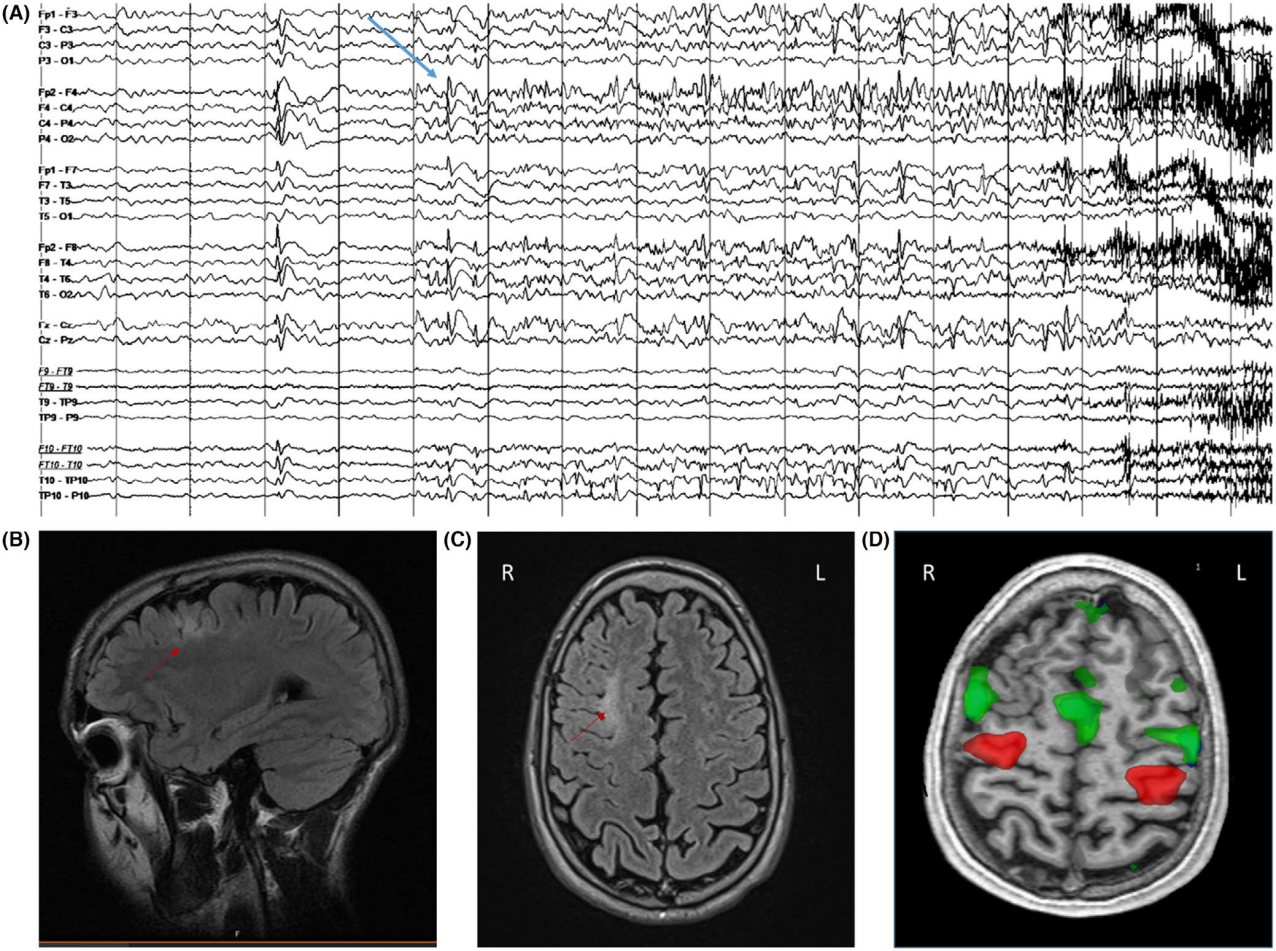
Phase I work‐up in a 24‐year‐old right‐handed male with a family history of epilepsy in his paternal uncle and nephew. Epilepsy onset was at age 7 years, initially with focal impaired consciousness seizures during childhood characterized by left‐sided facial twitching and leftward gaze deviation which no longer occurred after the age of 9. Subsequently, all seizures were focal to bilateral tonic–clonic, occasionally preceded by an ill‐defined aura described as full body discomfort, occurring monthly. Seizures were recorded as episodes typically occurring out of sleep and rarely preceded by an ill‐defined aura (patient alerts observer or pushes the alarm, however, is unable to explain his symptoms) and hyperventilation, with subsequent versive head turn to the left, gaze deviation to the left, tonic posturing with Figure 4 (flexion of the right upper extremity, extension of the left upper extremity), followed by bilateral clonic activity with postictal confusion. (A) Clinical onset typically occurred 8–10 s after electrographic onset, with first ictal changes on scalp EEG characterized by spike and slow wave discharges followed by 10–12 Hz repetitive spiking over the right frontal region (with maximum negativity over F4 > Fp2 > Fz) (arrow, 16 s, 1–70 Hz bandpass, bipolar longitudinal montage with subtemporal chains). (B, C) 3 T Brain MRI showed T2/FLAIR hyperintense signal and blurring of the gray‐white matter junction at the level of right superior frontal sulcus. (D) Functional MRI showed left dominant expressive and receptive speech with accessory expressive speech within the right frontal lobe 1–2 cm inferior and lateral to the lesion (green), and left hand motor function within 1 cm posterior and lateral to the right frontal lobe lesion (red). Neuropsychological evaluation results were not lateralizing or localizing. The primary hypothesis for the epileptogenic zone was a right frontal focus based on the scalp EEG seizure onset pattern, lesion within the right superior frontal lobe, and early elementary motor manifestations. Intracranial EEG monitoring was recommended to identify margins for potential surgical resection and for motor mapping given proximity of the lesion to the hand motor region (Figure 14). His 5‐SENSE score suggested that SEEG is likely to identify a focal seizure onset zone. Computation of his Specific Consistency Score suggests a score of 7 (grade B) which indicates that his data were partially consistent and further supports the decision for invasive monitoring.
